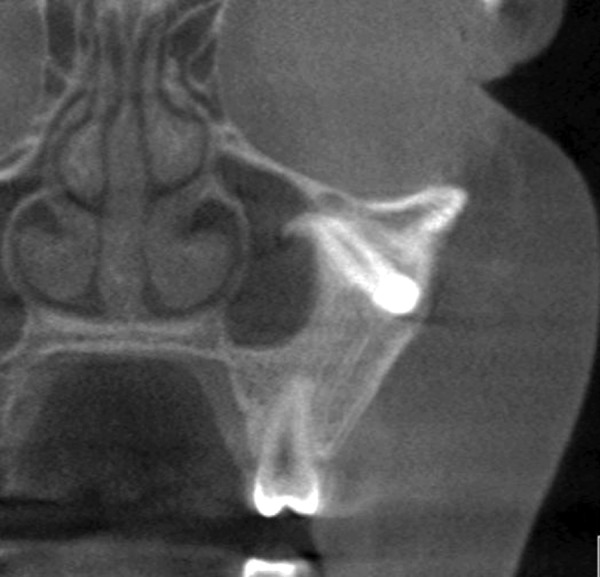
Cone beam CT image showing the position of the ectopic 23 in the zygomatic bone
